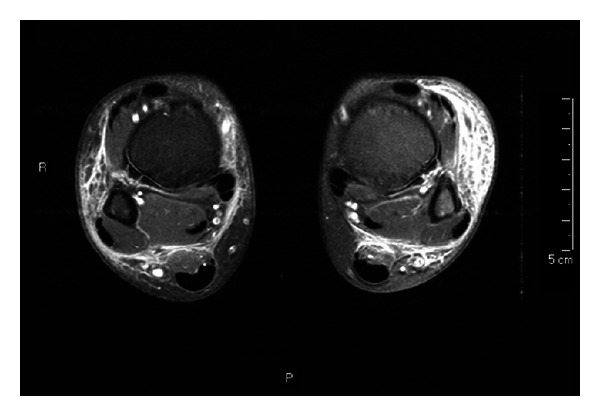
Magnetic resonance imaging of ankles and legs (coronal T2 and axial T1) showing bilateral cellulitis. Images show an extensive signal alteration of the subcutaneous soft tissues in both legs with dishomogeneous enhancement after contrast, consistent with an inflammatory involvement (cellulitis). A bilateral small intra-articular collection can be seen, without significant enhancement after contrast. Neither alterations in muscles and tendons, nor signs of osteomyelitis are detectable.
